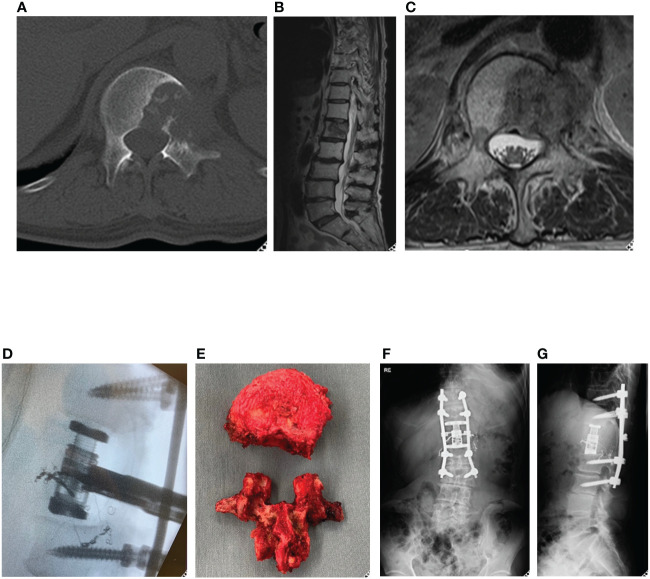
Representative case (total en bloc spondylectomy). 70-year-old man, primary left lung cancer, L1 Spinal metastasis. Axial CT (A) and T2-weighted MRI sagittal (B) and axial (C) revealed osteolytic lung cancer metastasis in the left pedicle of the L1 vertebral body. A single posterior approach total spondylectomy was performed (D). For reconstruction of the anterior column, an expandable cage was inserted posteriorly, followed by posterior fixation (E-G). total en bloc spondylectomy (TES).
